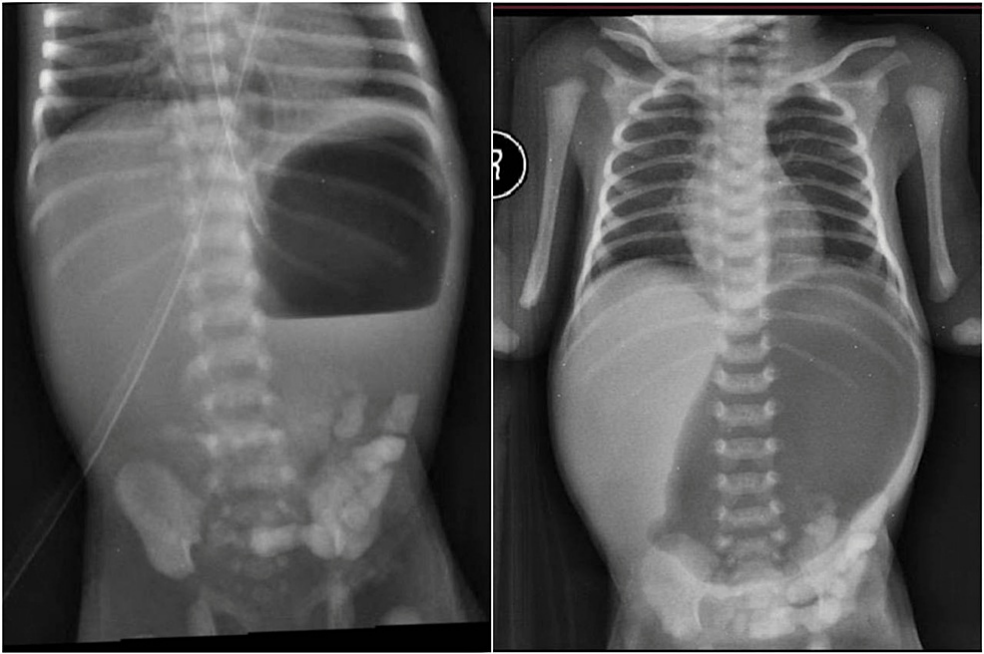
Plain X-ray showed a huge, dilated stomach.
